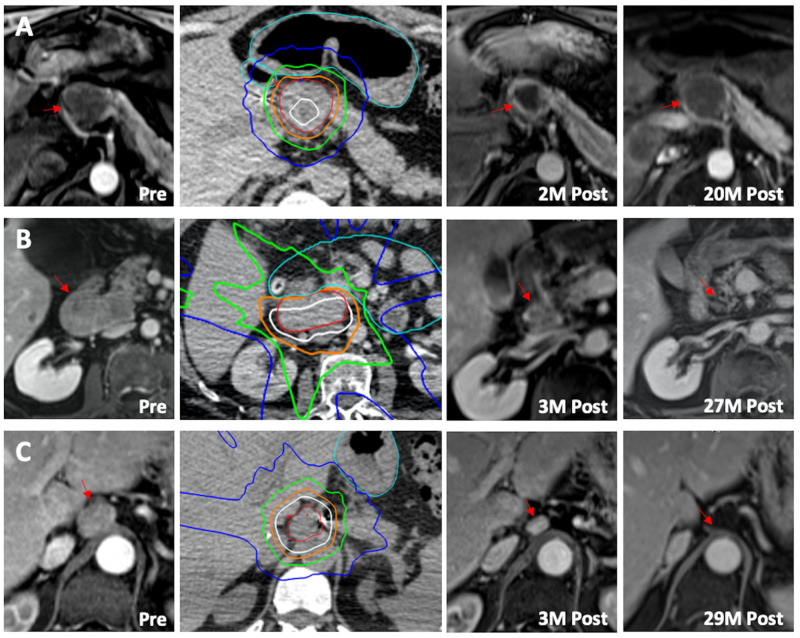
Radiographic response to SBRT SBRT treatment in three patients (A, B, and C) with isolated LNM from HCC, with pre-treatment MRI (1st column, red asterisk = LNM), treatment planning scan (2nd column, red outline = gross tumor volume, light blue = bowel avoidance structure, dark blue = 10 Gy, green = 20 Gy, orange = 30 Gy, white = 40 Gy), initial post-treatment MRI (3rd column), and long-term follow-up MRI (4th column). Patient A had recurrence in the liver at two months and stable disease in the treated node at 20 months, whereas patients B and C remained without evidence of disease recurrence at 27 months and 29 months, respectively.
